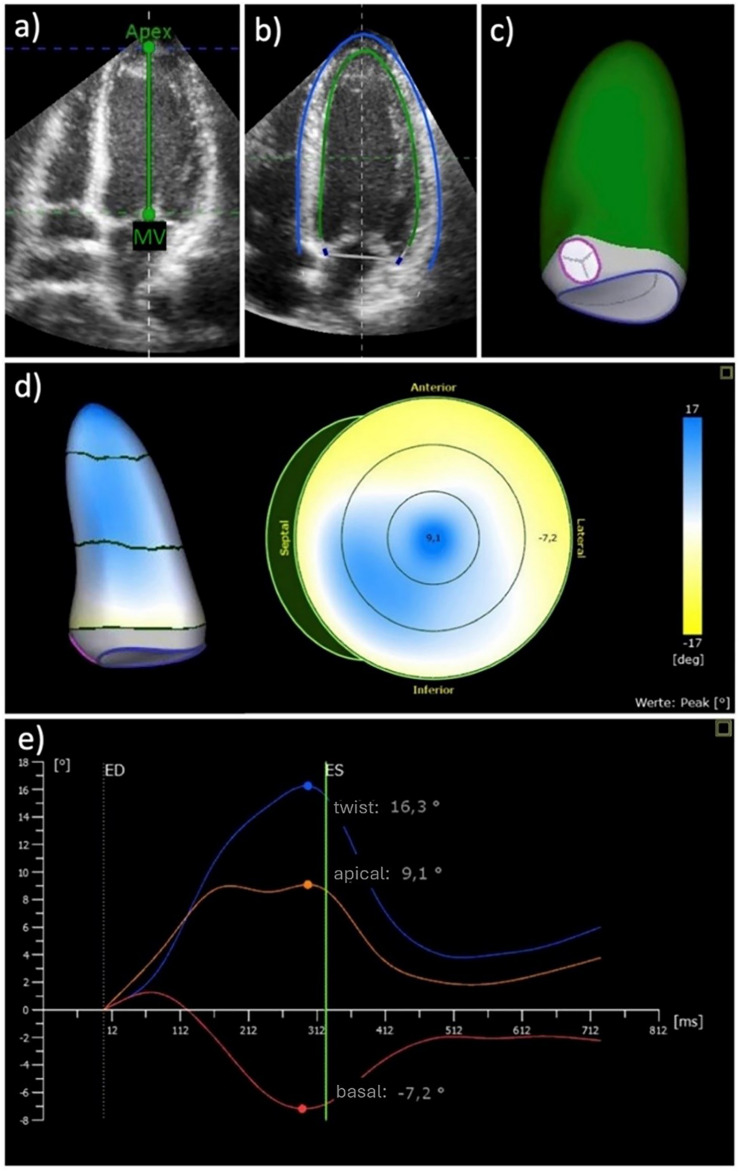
Image analysis and determination of left ventricular (LV) twist through 3D echocardiography. (a) Marking of the mitral valve (MV) and apex of the LV. (b) Contour of the LV (blue line), the endocardium (green line) and marking of the mitral valve (blue dots). (c) 3D reconstruction of the LV. (d) Visualisation of the apical (blue) and basal (yellow) rotation of the LV. (e) LV rotation during the cardiac cycle. Orange line: apical rotation, red line: basal rotation, ED: end-diastole, ES: end-systole. Twist (blue dot): the sum of the absolute values of the maximum apical (orange dot) and basal (red dot) rotation.
